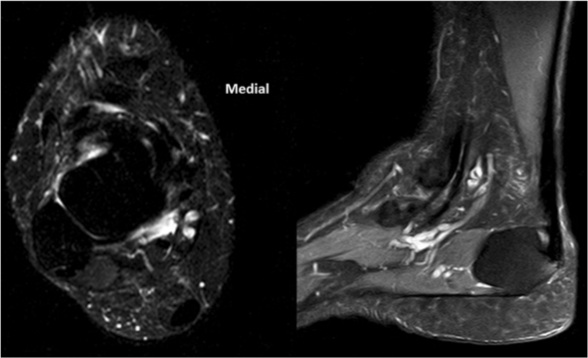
MR images obtained 85 days after surgery. Disappearance of tendon gap appears in T2 with fat sat.
